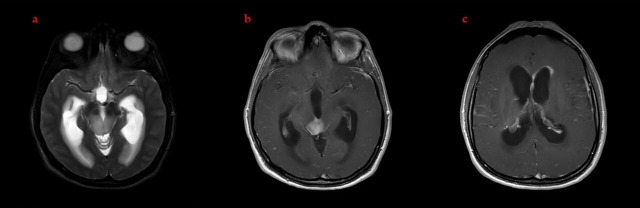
Diagnostic brain MRI findings at presentation. (a) Axial T2-weighted image demonstrating a hyperintense mass at the level of the tectal plate–mesencephalon with resultant hydrocephalus secondary to aqueductus cerebri obstruction. (b) Axial post-contrast T1-weighted image showing homogeneous enhancement of the lesion. (c) Axial post-contrast T1-weighted image demonstrating ependymal enhancement along the frontal horn of the left lateral ventricle and the interventricular septum, consistent with tumor spread.
